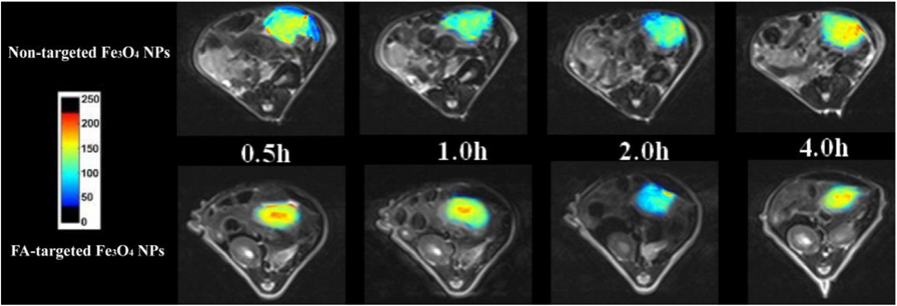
In vivo MR imaging of intraperitoneal tumor after intravenous injection of FA-targeted Fe_3_O_4_ NPs or non-targeted Fe_3_O_4_ NPs (0.6 mg Fe) at different time points
